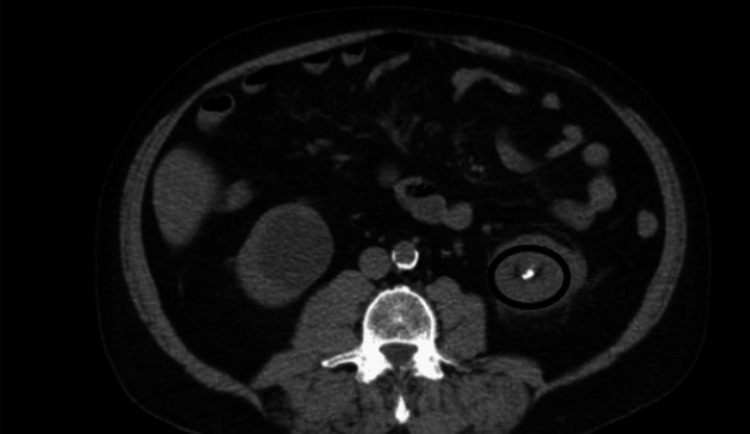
CT scan abdomen axial view: showing a white hyperdense calcified lesion representing a renal stone in left kidney. Mild left hydronephrosis and hydroureter attributed to a 0.3 cm calculus lodged within the distal left ureter.
